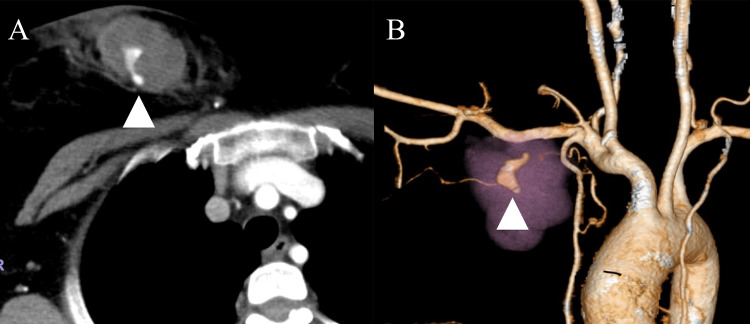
Computed tomography revealed a right anterior thoracic hematoma with extravasation (A, horizontal axial view (white arrow heads), and B, three-dimension construction view (white arrow heads))
